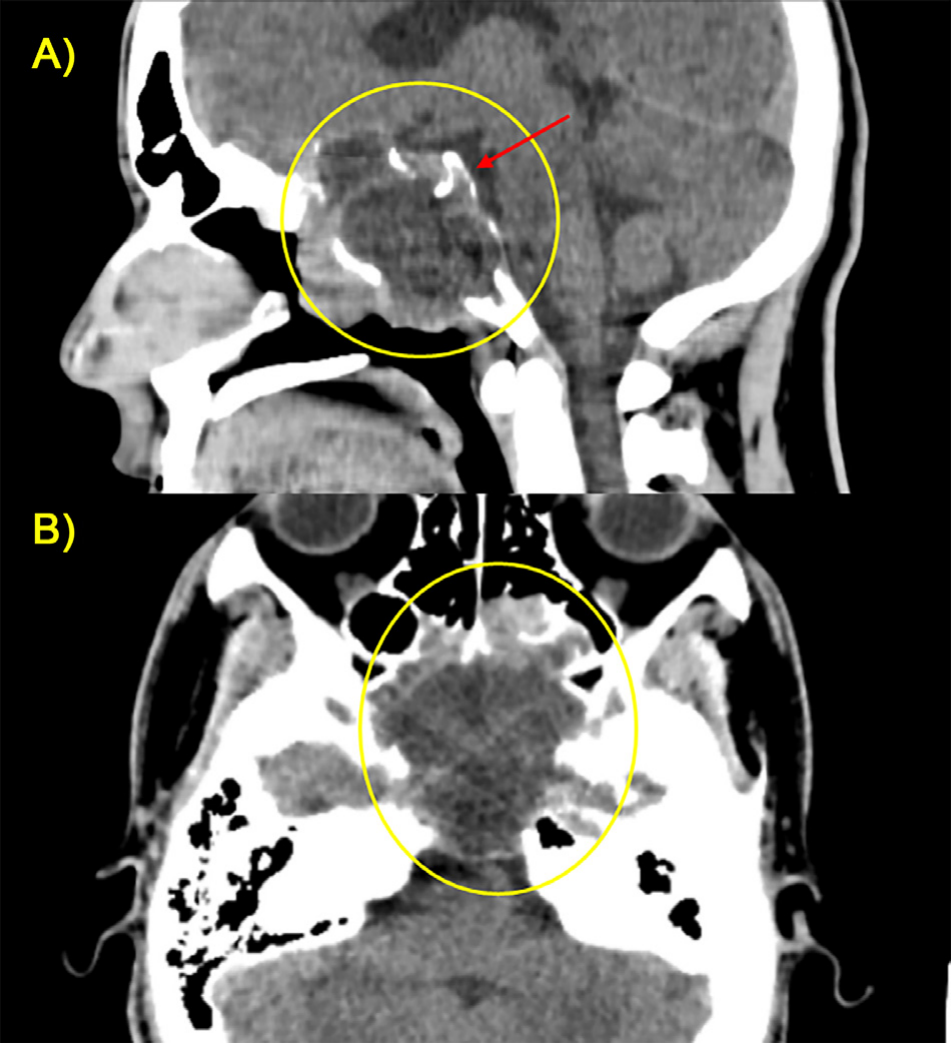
(A, B) Sagittal (A) and axial (B) CT scan without contrast administration showed with the soft tissue window a large heterogeneous mass (yellow circle) with marginal sclerosis (red arrow).
